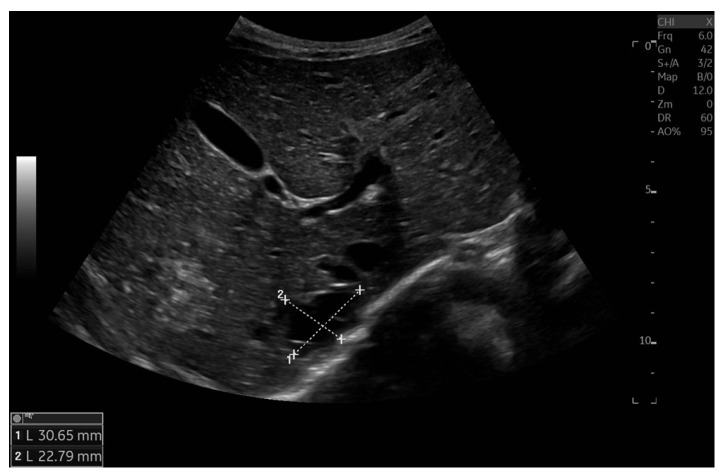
Ultrasonography of the liver with the largest cluster of cysts.
